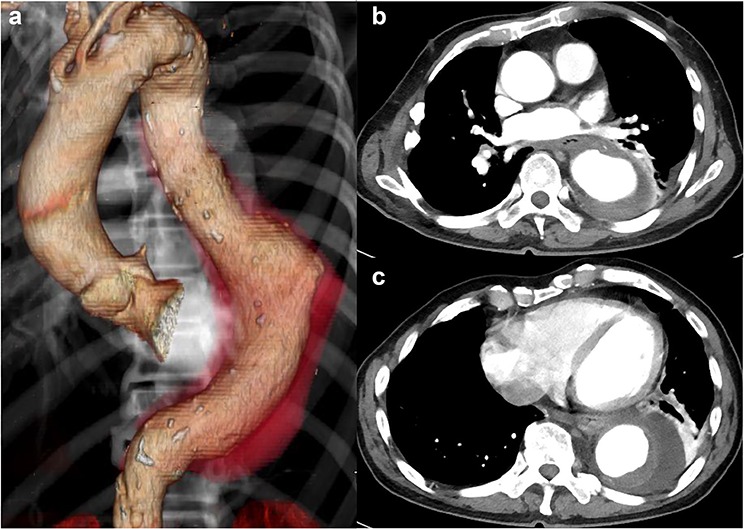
CT shows a descending aortic aneurysm (a), 50 mm in diameter (b), surrounded by an effusion (c).
